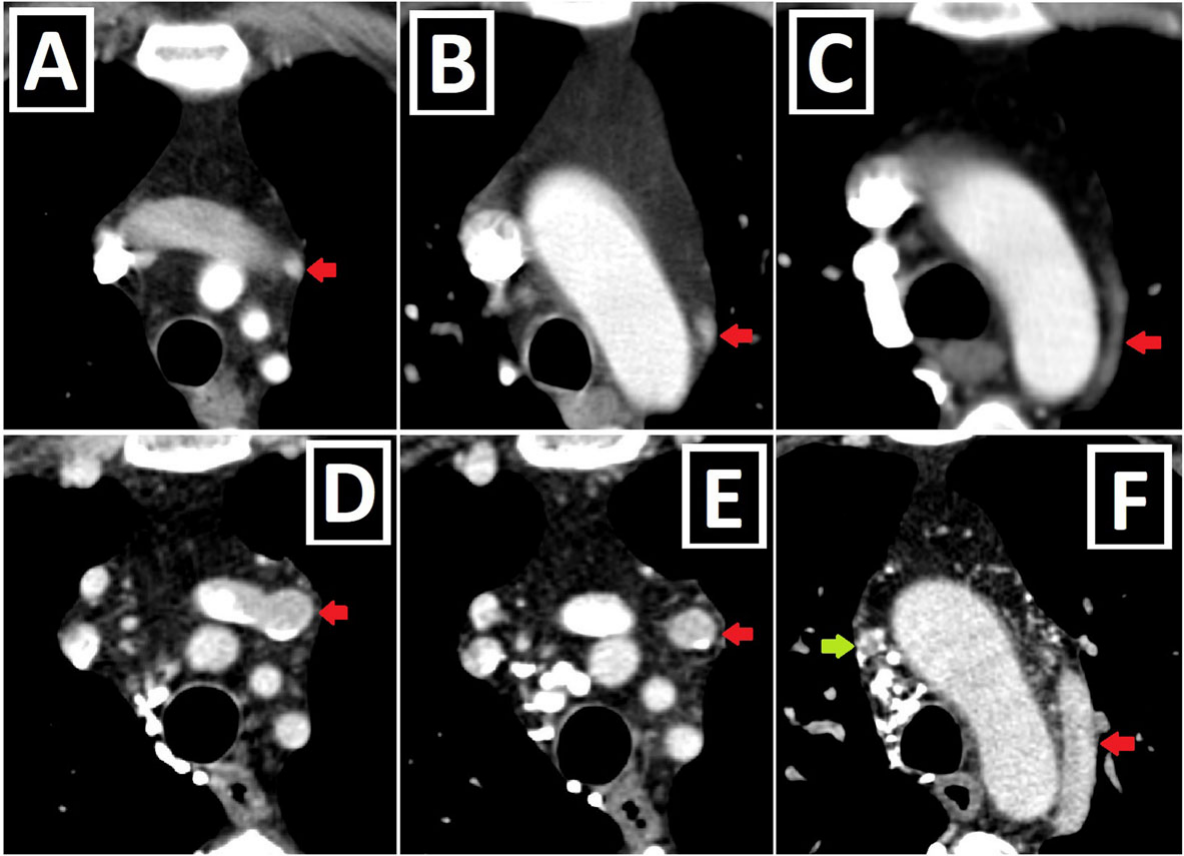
Left superior intercostal vein. a–c Axial CT images depict a thin-caliber LSIV (red arrows) in a healthy patient. d–f After developing SVC occlusion (green arrow, f), axial CT images show multiple mediastinal venous collaterals and markedly enlarged LSIV (red arrows) in the same patient
